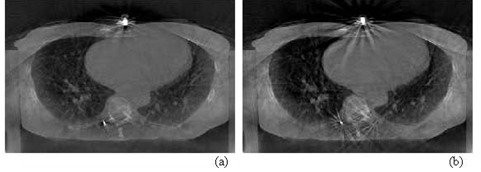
CBCT transversal images of a patient lung reconstructed from cone‐beam projections before (a) and after (b) motion correction.
